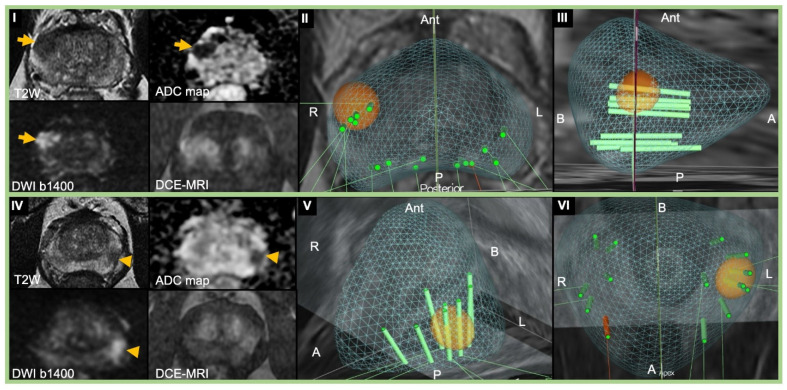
Representative images of 3D-TRUS/MRI fusion-guided transperineal and transrectal prostate biopsy. (I–III) A 75-year-old man with PSA 9.48 ng/mL who underwent 3D-TRUS/MRI fusion-guided TP prostate biopsy under local anesthesia with Gleason Grade Group 5 prostatic adenocarcinoma detected on target biopsy without any complications. (I) Pre-biopsy multiparametric MRI showing a 1.2 cm PI-RADS 4 lesion (arrow) in the right mid-anterior peripheral zone. The lesion was moderately hypointense on T2W, focal markedly hypointense on the ADC map, markedly hyperintense on high b-value DWI, and showed focal early enhancement on DCE. (II) Transverse and (III) right sagittal view of 3D-TRUS/MRI fusion-guided TP prostate biopsy (green cores) cartography. Blue mesh is the contour of the prostate. Target (orange sphere) was assigned on right mid-anterior peripheral zone PI-RADS 4. (IV–VI) A 62-year-old man with PSA 5.98 ng/mL who underwent 3D-TRUS/MRI fusion-guided TR prostate biopsy under local anesthesia with Gleason Grade Group 3 prostatic adenocarcinoma detected on target biopsy without any complications. (IV) Pre-biopsy multiparametric MRI showing 0.9 cm PI-RADS 4 lesion (arrowhead) in the left mid posterior peripheral zone. The round-shaped lesion was moderately hypointense on T2W, focal markedly hypointense on the ADC map, markedly hyperintense on high b-value DWI, and showed focal early enhancement on DCE. The segmentation is the same as TP biopsy. (V) Left sagittal view and (VI) coronal view of 3D-TRUS/MRI fusion-guided TR prostate biopsy (green cores) cartography. Target was assigned on left posterior apex peripheral zone. T2W, T2 weighted; ADC, apparent diffusion coefficient; DWI, diffusion-weighted imaging; DCE, dynamic contrast-enhanced; A, apex; B, base; R, right; L, left; Ant, anterior; P, posterior; PSA, prostate-specific antigen; MRI, magnetic resonance image; PI-RADS, Prostate Imaging Reporting and Data System; 3D, three-dimension; TRUS, transrectal ultrasound; TP, transperineal; TR, transrectal.
